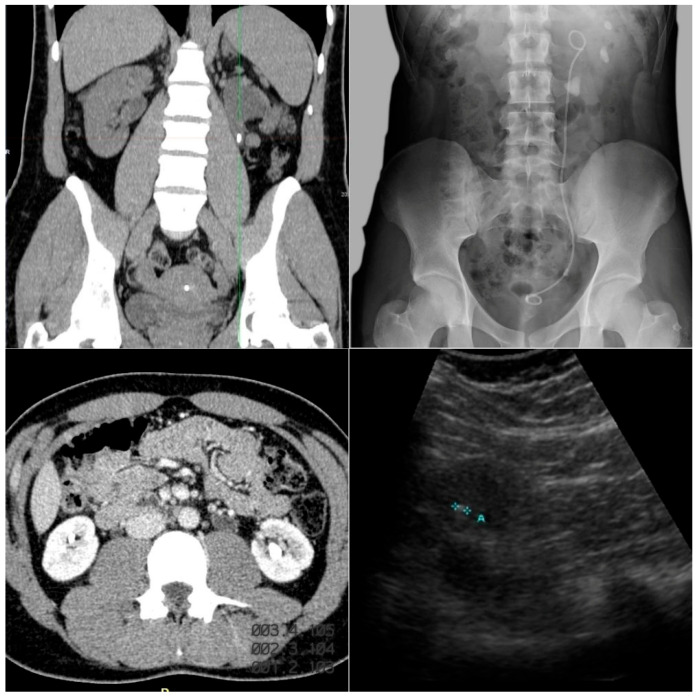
Representative imaging of nephrolithiasis in our case series of patients with primary hyperoxaluria in Hospital Universitario La Paz. Computed tomography (left images): coronal section (top) and axial section (bottom) showing hyperdense foci within the urinary system, consistent with urolithiasis. Plain abdominal radiograph (top right): a double J ureteral stent is identified on the left side, associated with radiopaque calcified densities projected onto the renal silhouette, consistent with lithiasis. Ultrasound (bottom right): hyperechoic focus with posterior acoustic shadowing, a characteristic finding of urinary lithiasis.
